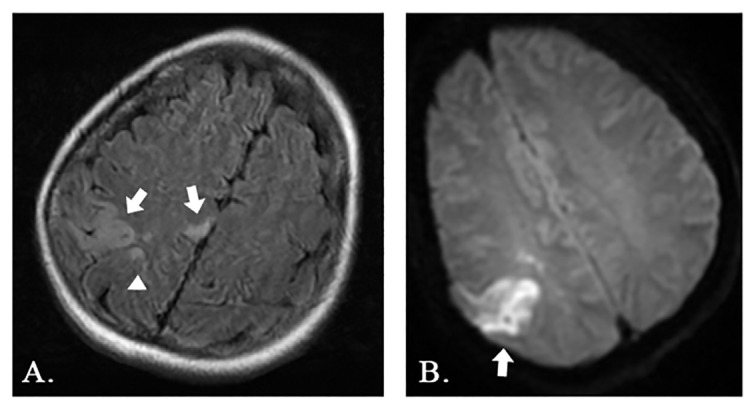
Brain magnetic resonance imaging showing (A) multiple foci of abnormal restricted diffusion predominantly involving the right parietal lobe (arrows) and right occipital lobe (arrowhead), and (B) hyperintense signal of the right parietal lobe on axial T2 fluid attenuated inversion recovery (FLAIR) sequence (arrow).
